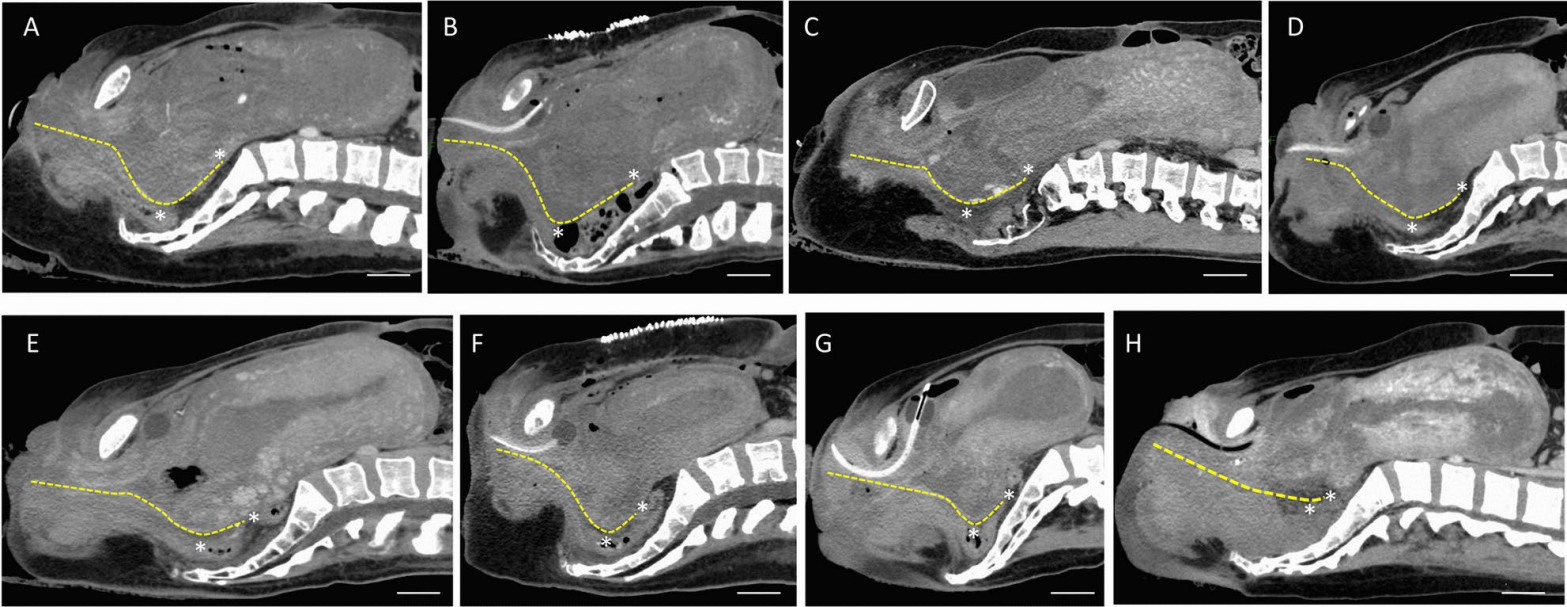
Displacement of posterior vaginal wall by clot accumulation in the birth canal. Sagittal images from the late phase of dynamic CT scans in patients transported for the management of severe primary postpartum hemorrhage (PPH) (A–H). Cases A and C showed contrast extravasation, with Case A involving a rupture of the lower uterine segment and Cases C and H resulting from vaginal lacerations. The remaining cases were due to uterine atony. The posterior vaginal walls are indicated by yellow dashed lines, and the asterisk marks the most dorsal aspect of the vaginal canal and the posterior fornix. The posterior vaginal wall was displaced dorsally due to the accumulation of blood clots in the birth canal (A–G). The distances between the asterisks, which may indicate the degree of upper vaginal extension, were as follows: A: 7.0 cm, B: 7.0 cm, C: 4.7 cm, D: 4.2 cm, E: 3.7 cm, F: 3.3 cm, G: 3.3 cm, and H: 0.9 cm. The scale bar indicates 4.0 cm.
